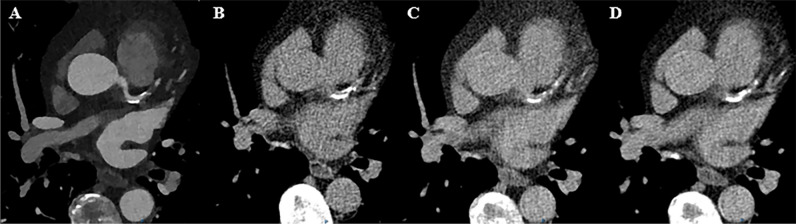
Calcium scoring from the photon-counting CT for a patient with calcifications in the LAD. A Contrast-enhanced CCTA. B Standard non-contrast imaging for calcium scoring (CAC = 461.1). C Virtual non-contrast reconstruction (CAC = 179.5). D Virtual non-iodine reconstruction (CAC = 538)
